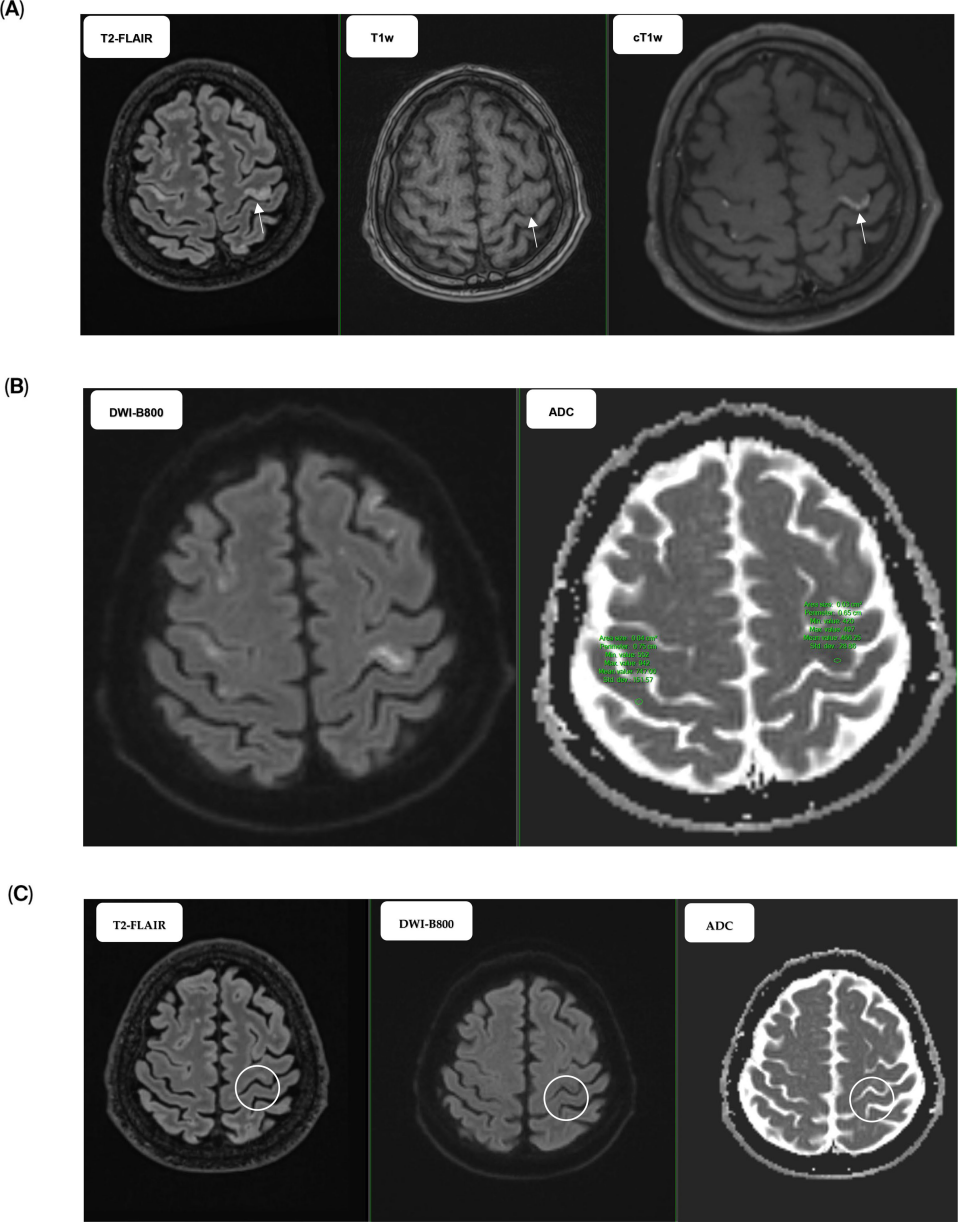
Secondary vasculitis following the administration of checkpoint inhibitors. (A) Brain MRI on February 14, 2025. Multiple T2-FLAIR hyperintense lesions in the supratentorial white matter and bilateral cortico-subcortical regions, with the most pronounced lesions in the frontoparietal region and relative sparing of the temporal lobes (arrow). No significant signal changes (arrow) on non-enhanced T1w images. Post-contrast T1w-black blood demonstrates cortical enhancement (arrow), indicating blood–brain barrier disruption. (B) Diffusion restriction is supported by objective high signal on DWI (b800) and ADC measurements demonstrating decreased ADC values within the lesions relative to normal-appearing contralateral parenchyma. The corresponding reduction is visible in the provided ADC map and ROI analysis. (C) Brain MRI on June 21, 2025. Follow-up MRI demonstrates regression of previously described T2-flair lesions (white circle) with restricted diffusion on DWI/ADC (white circle) with no new abnormalities. Contrast agent was not administered due to impaired renal function; therefore, assessment of enhancement was not possible.
